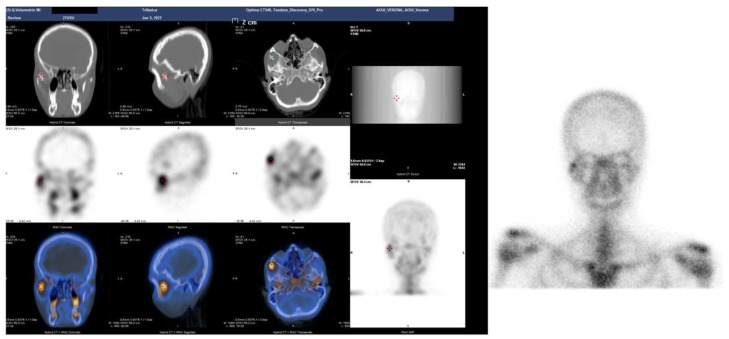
SPET-CT showing bone reworking in correspondence to the joint between the jaw and the right zygomatic bone compared to the contralateral homologous site.
